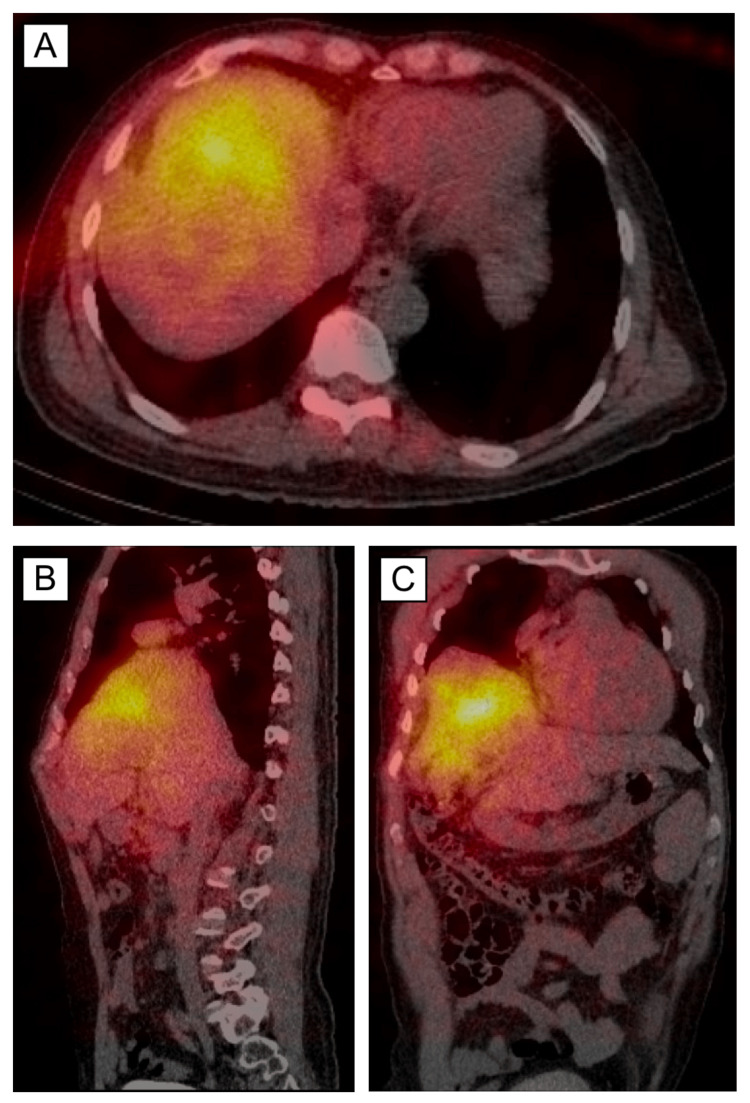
SPECT CT showing Y-90 microembolic microsphere distribution. (A/B/C) Axial, sagittal, and coronal planes showing Y-90 microembolic microspheres that are localized primarily to the liver area of interest where the HCC resides. SPECT: single-photon emission computed tomography; HCC: hepatocellular carcinoma
